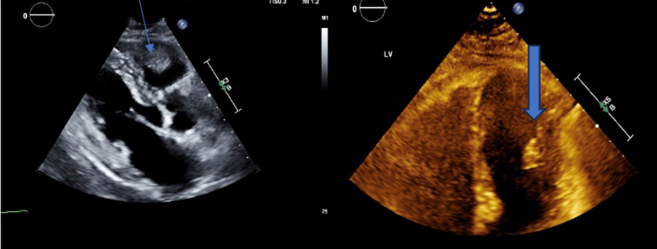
Findings on Transthoracic Echocardiography Cardiac echocardiography showing a 3.5-cm mass on the free wall of the right ventricle (thin blue arrow) and a 1.46-cm mass attached to the anterolateral papillary muscle in the left ventricle (thick blue arrow).
